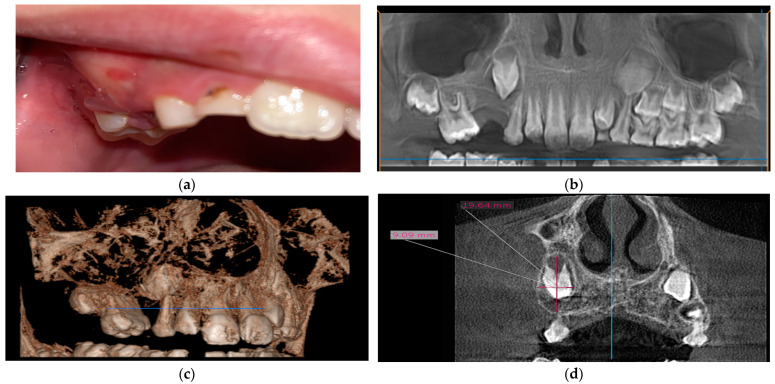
Case 1’s initial preoperative images: (a) intraoral view; (b) CBCT-panoramic view; (c) CBCT reconstruction; (d) cross-sectional view with measurements.
